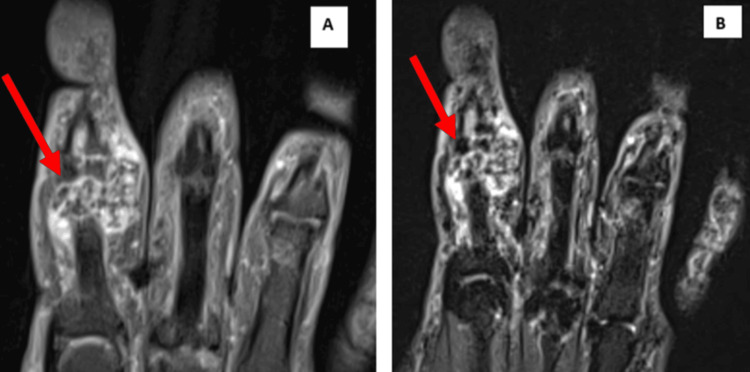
Proton density fat-saturated MRI sequence (PDFS) and short tau inversion recovery (STIR) images of the lesion near the proximal interphalangeal joint of the index finger 2A: Proton density fat-saturated MRI sequence (PDFS) and red arrows show heterogeneously hyperintense areas. 2B: Short tau inversion recovery (STIR) and red arrows show the heterogeneously hyperintense area with hypointense areas within.
